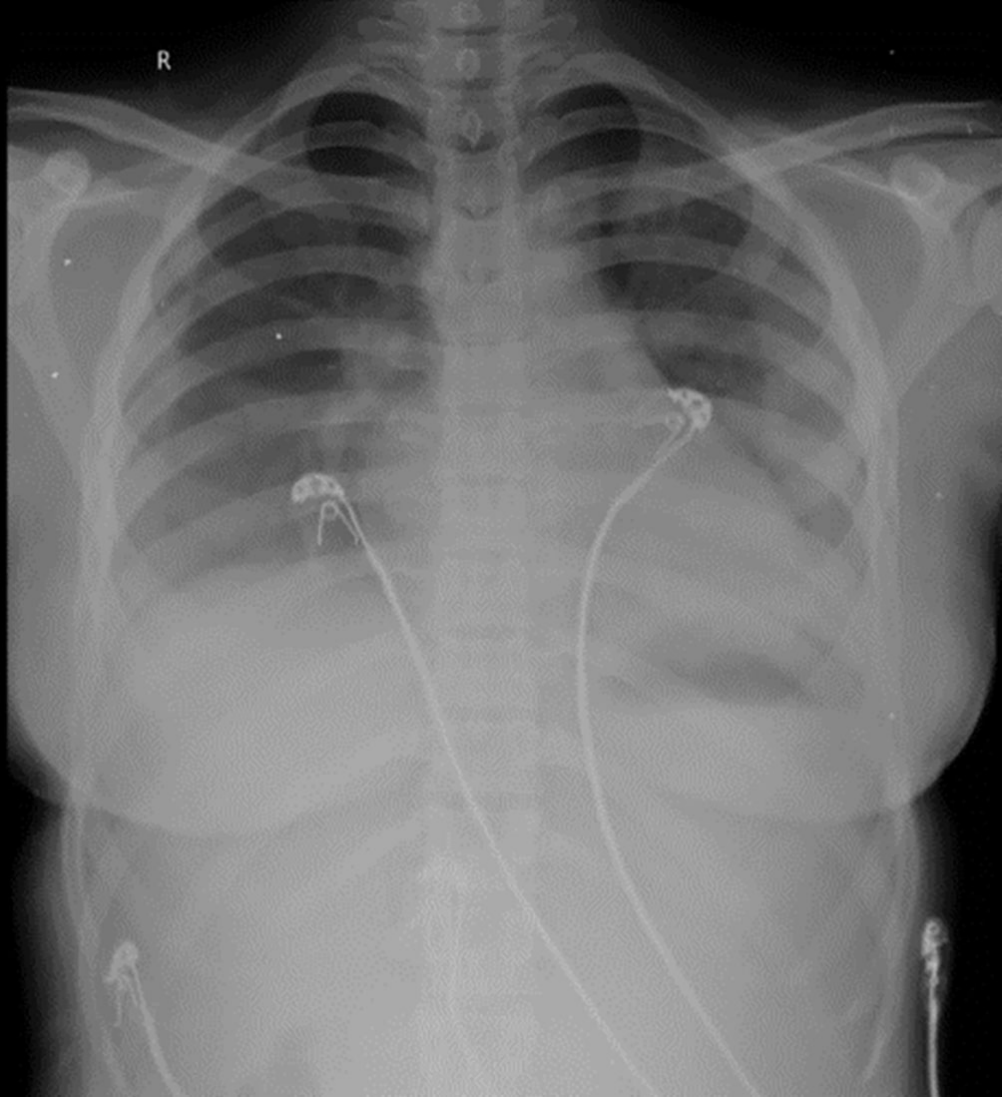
Chest radiograph PA view, day 8.
